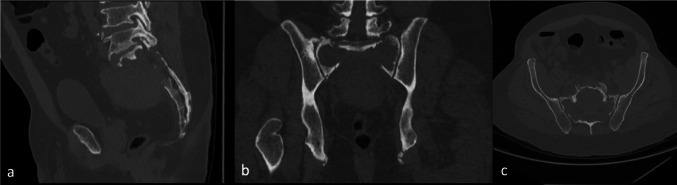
Preoperative CT spine (A sagittal; B coronal; C axial) mixed lytic destructive lesion involving bilateral sacral body with nondisplaced pathological fracture
